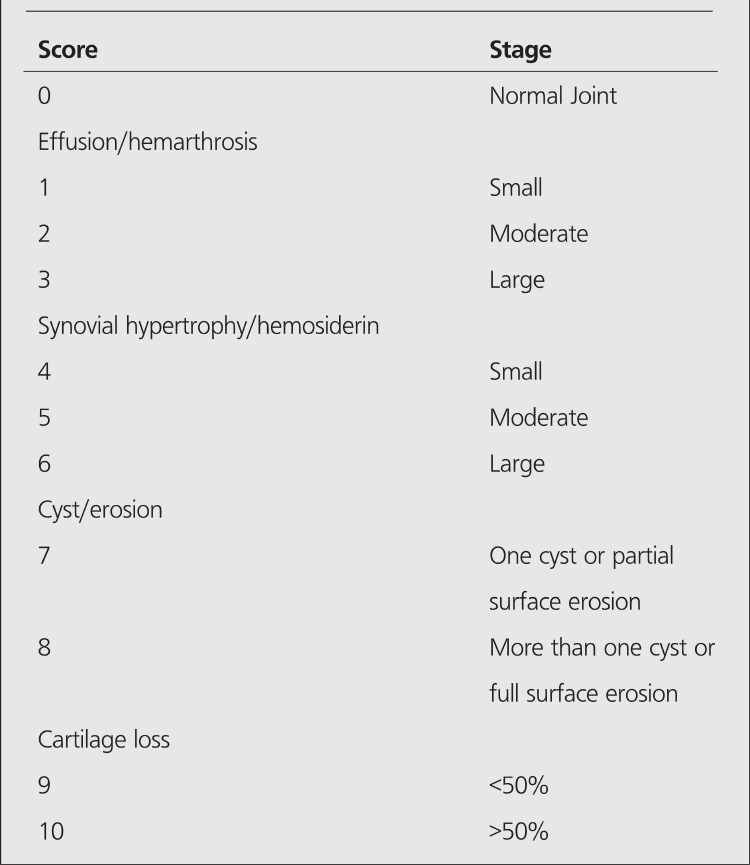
The Denver MRI scale classifying the arthropathy indifferent stages in relation to the most severe finding.The maximum score is 10
